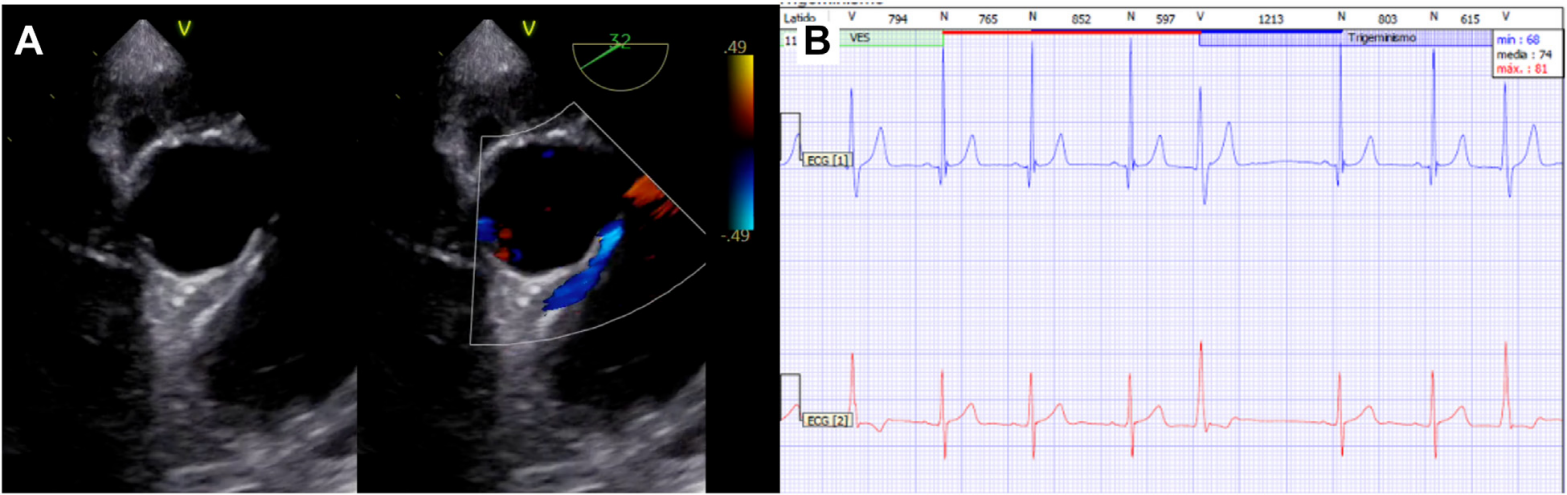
Transesophageal Echocardiogram and Holter Monitoring (A) Midesophageal short-axis view shows anomalous origin of the right coronary artery from the left aortic sinus with an interarterial course; the fixed narrow coronary lumen strongly suggests an intramural segment. (B) 24-hour Holter monitoring registered 8% monomorphic premature ventricular contractions, in isolation and trigeminy.
